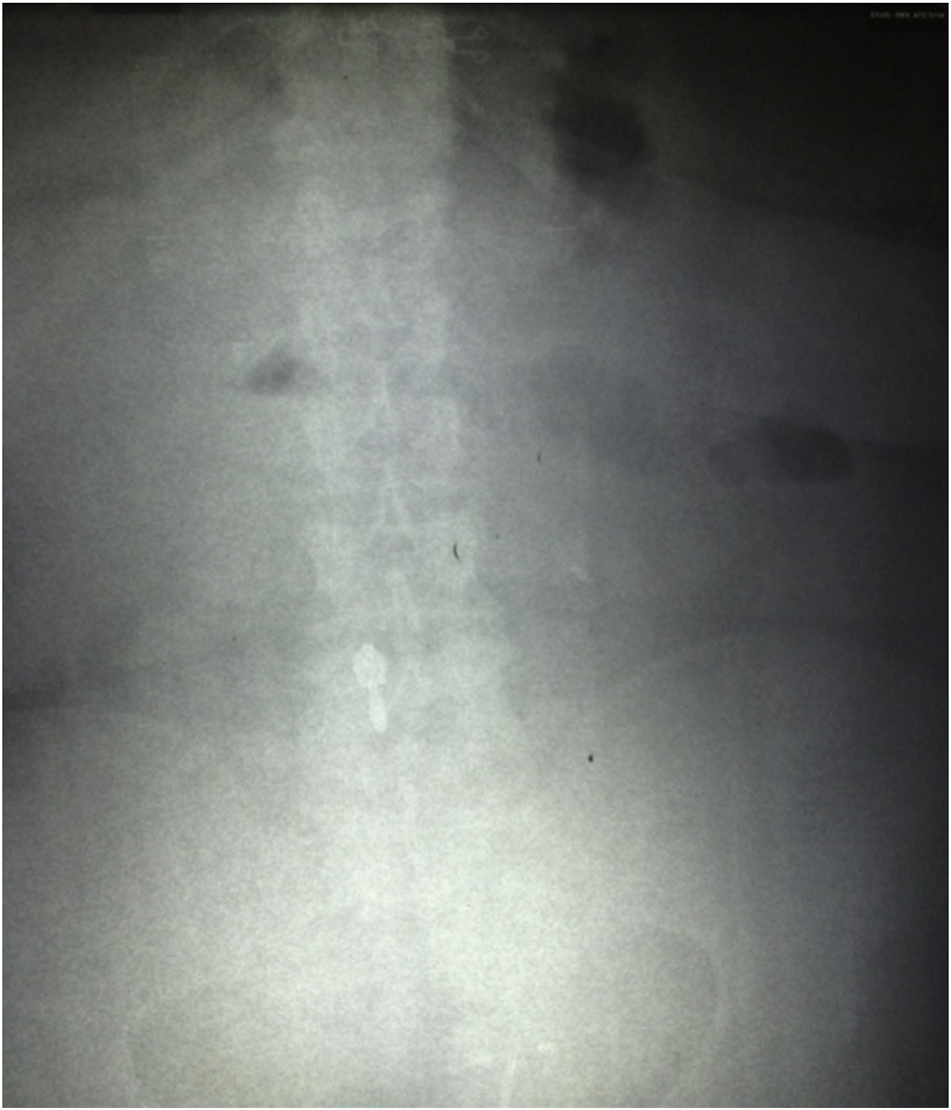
Upright abdominal radiograph revealed gas shadow in the stomach and duodenum.
